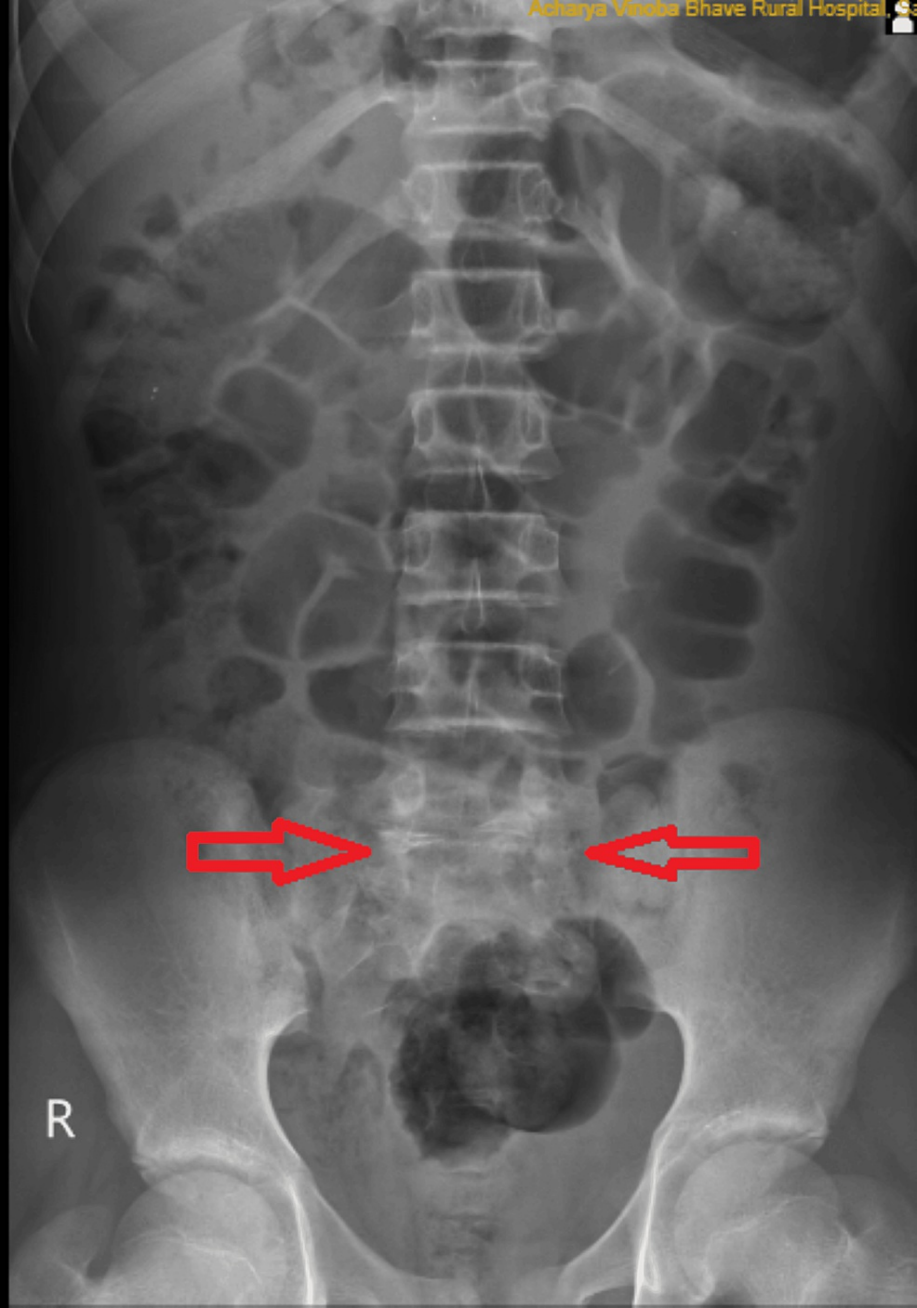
Anterior-posterior X-ray of the lumbar spine; initial injury X-ray at the time of presentation
